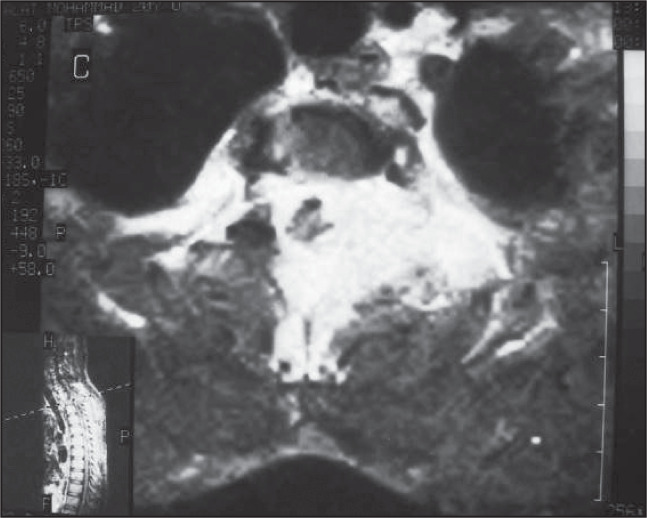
Axial T1-weighted gadolinium-enhanced magnetic resonance image showing dense enhanced paravertebral mass encasing the spinal canal, suggestive of hemangiopericytoma in a young man.
